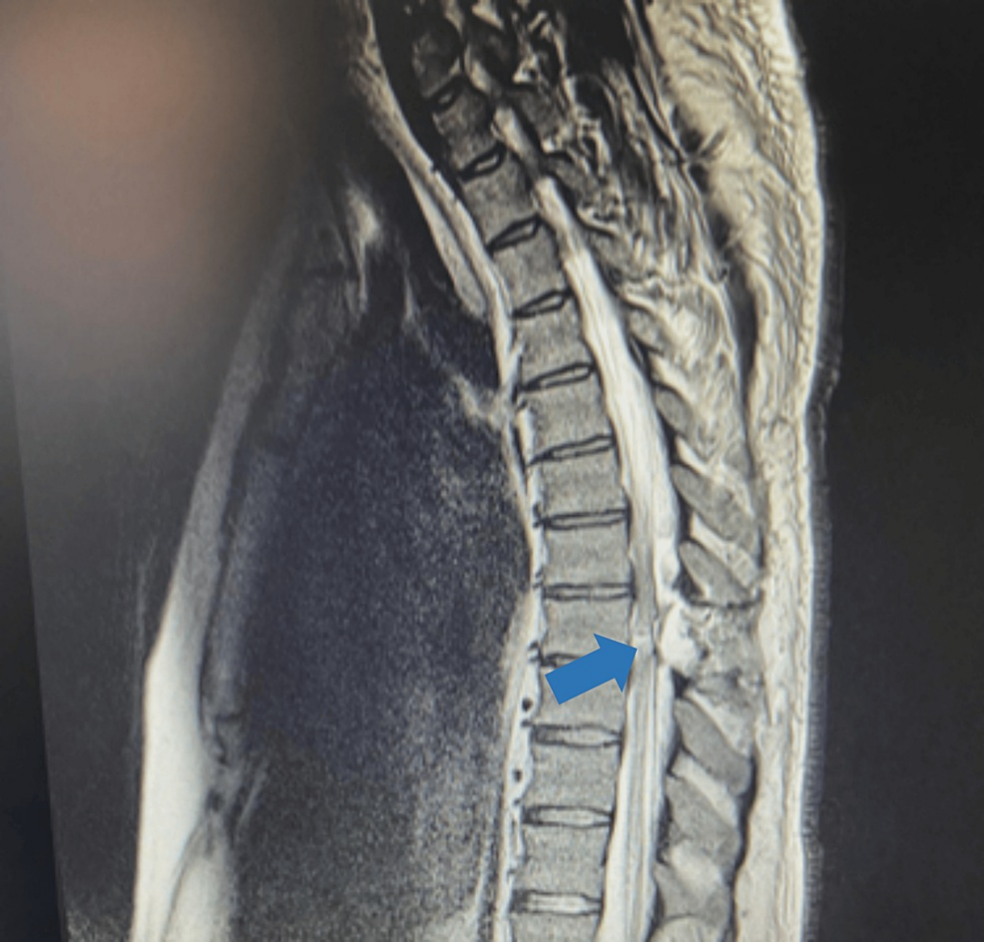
T2-weighted magnetic resonance imaging revealing an altered signal area in the right hemimedulla (blue arrow).
